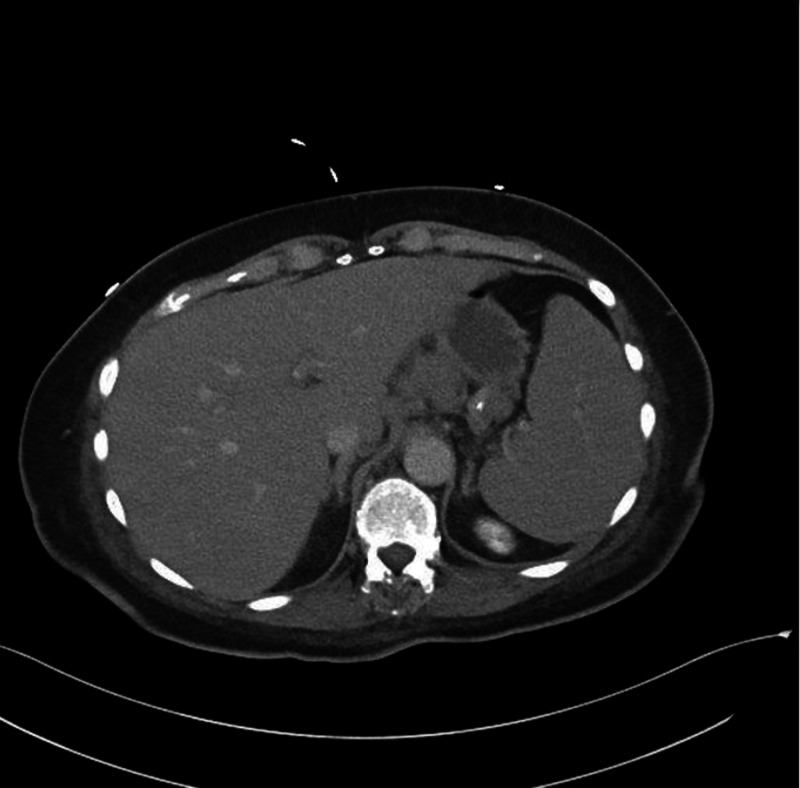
CT with IV contrast reveals normal appearing liver and moderate splenomegaly
